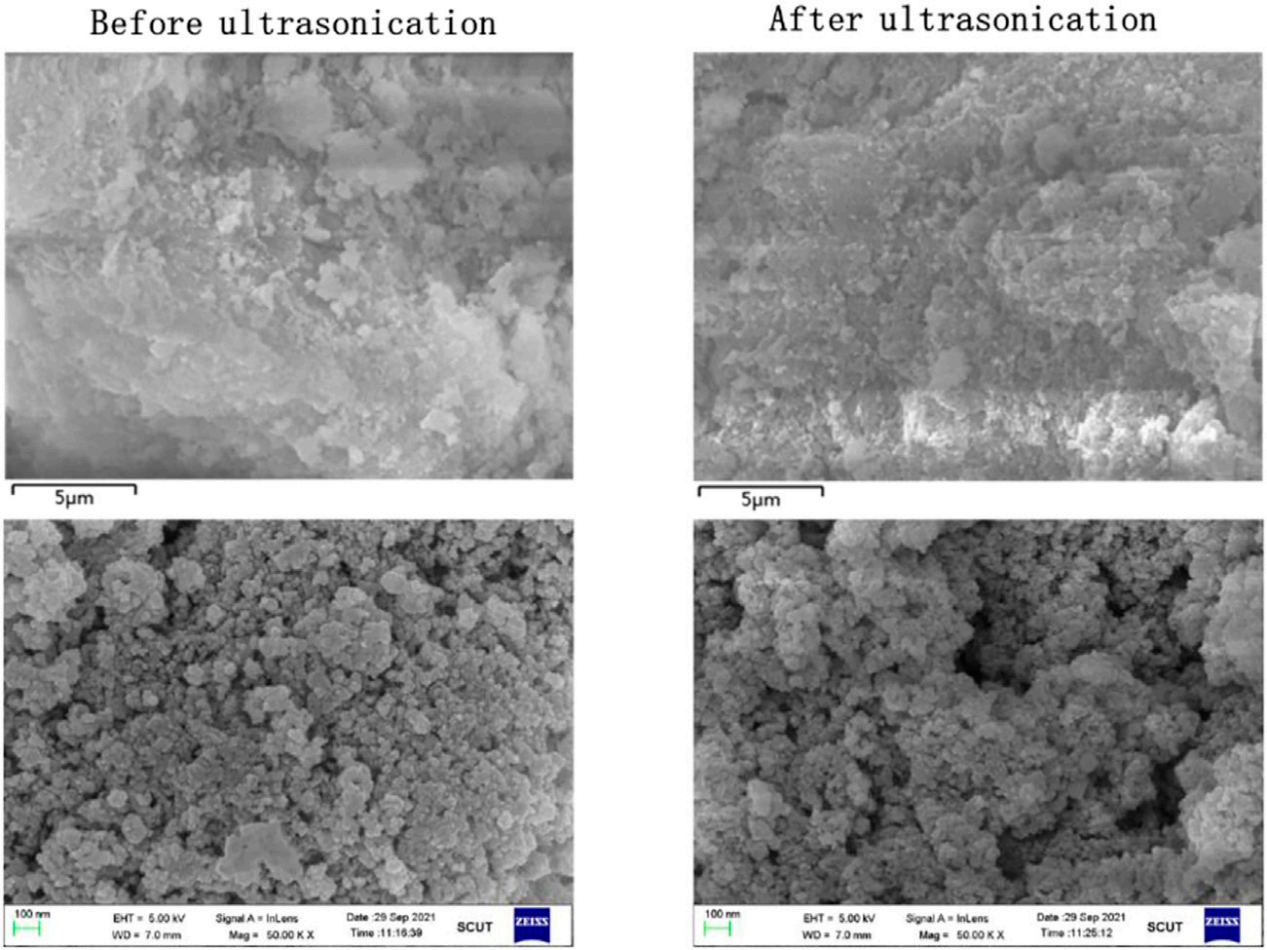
Topography change of complex sludge (EPS2) before and after ultrasonic treatment.
